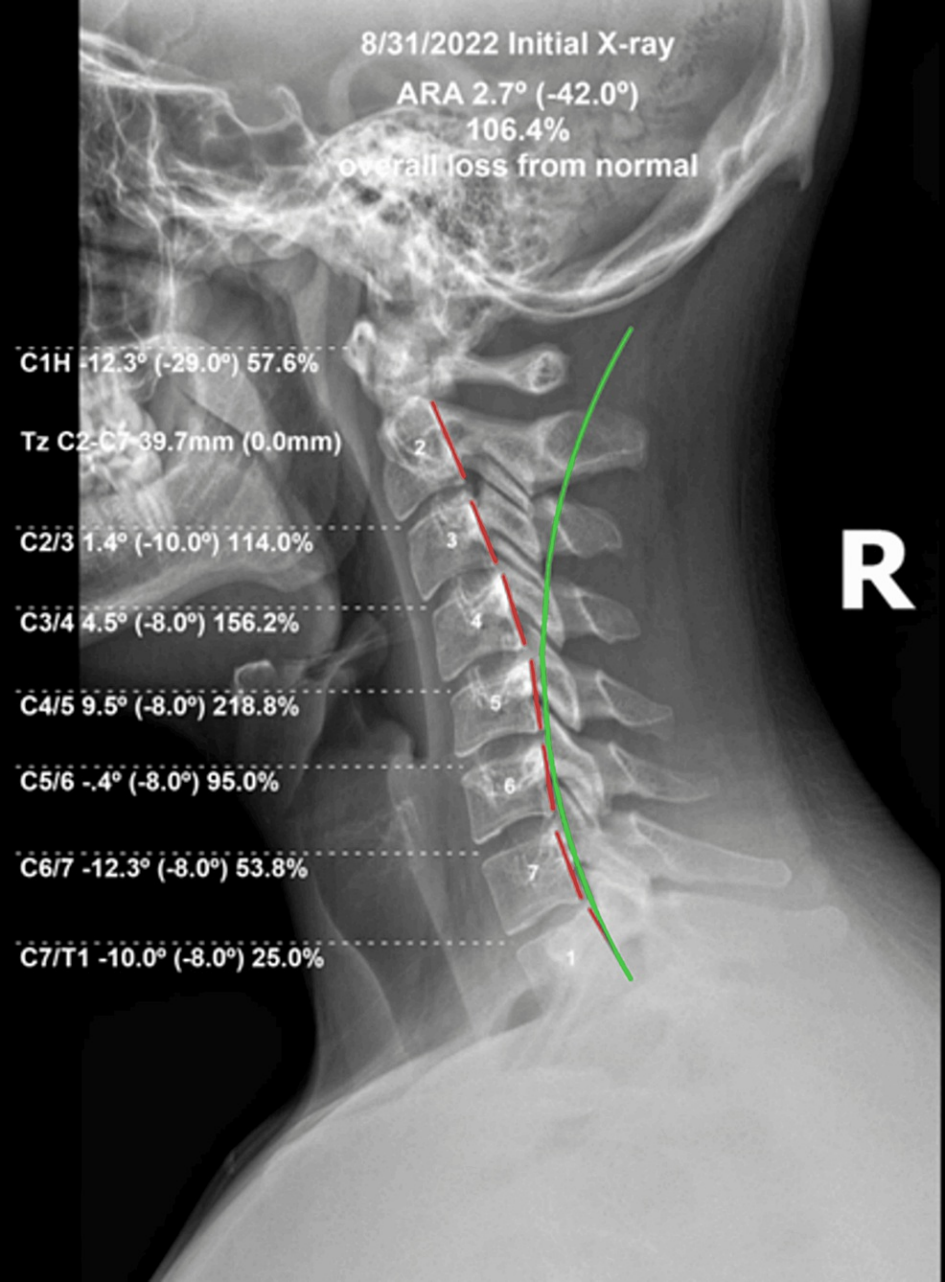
Upright, standing lateral cervical radiograph at the initial examination The ARA from C2 to C7 measures 2.7° compared to a normal range of -20° to -42°. The upper cervical spine is markedly anterior with a large abnormality visible. The green line represents normal lordosis, and the dashed red line demonstrates the posterior tangent of the segments as measured with the HPTM. The segmental angles reported on the left are the individual RRAs. These angles are the first derivative of the curvature of the column, and the greater the abnormality, the larger the stresses and strains generated on the tissues ARA: absolute rotation angle; HPTM: Harrison posterior tangent method; RRAs: relative rotation angles
